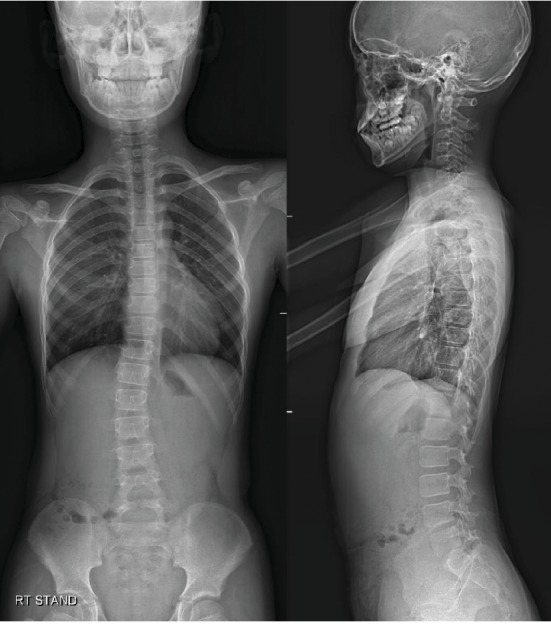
Whole spine plain radiograph. AP and lateral view at the initial visit, at July 9^th^, 2012.
